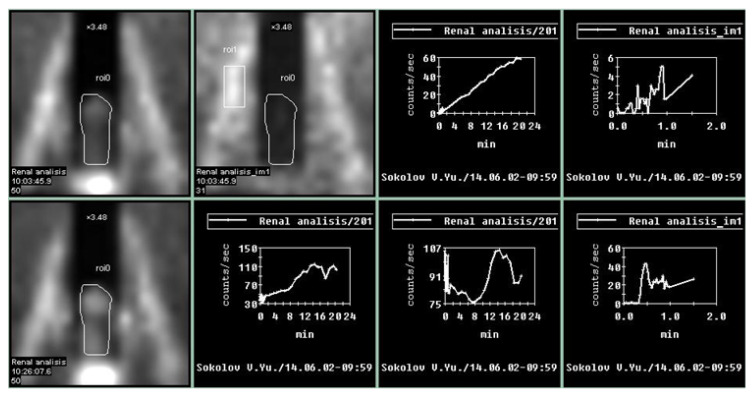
Normal parameters of the penile scintigraphy: arterio-organ transit time of the radiopharmaceutical drug is fast, at 3 s; the arterial and venous segments are clearly defined. An ascending segment is defined on the integral curve, with preserved phase oscillations without clearly expressed descending segments. On curves obtained after pharmacological stimulation, there is an increase in contrast accumulation activity up to 200%.
